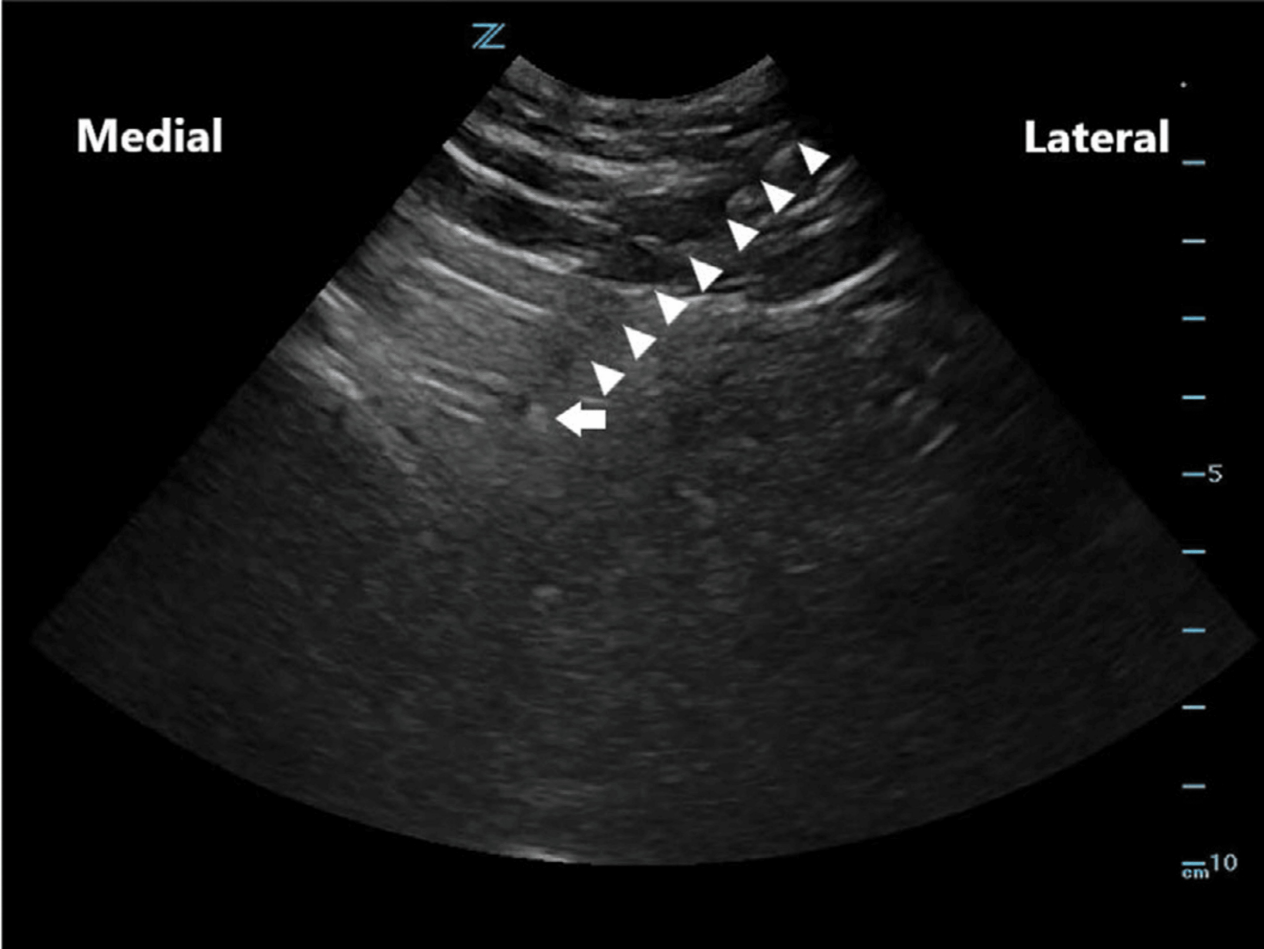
Needle visualization under ultrasound guidance. The needle is seen as it tracks through the tissues (white arrowheads) until the needle tip (white arrow) reaches the piriformis muscle [9].
